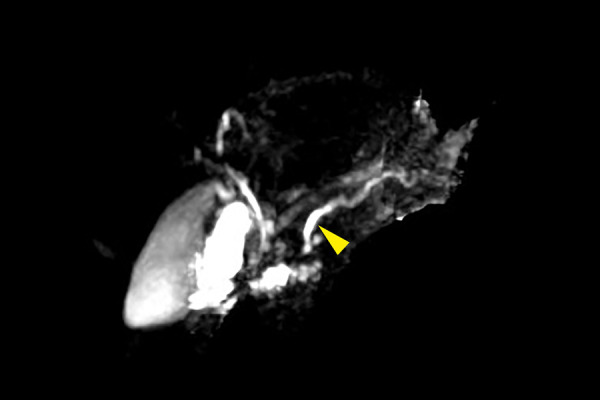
(Case 5): follow-up MRI/MRCP 4 months postoperatively shows mild dilation of the pancreatic duct, with a maximum width of approximately 2.3 mm (yellow arrowhead).
